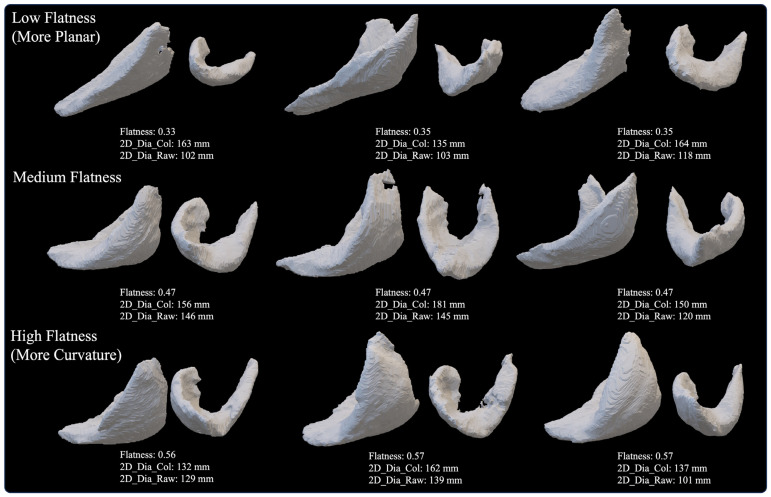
Representative Deltoid Muscle with Low (top row, more planar), Medium (middle row), and High (bottom row, more curvature) Values of Deltoid Shape Flatness for 3D Volumes Reconstructed from BONE Kernel CT Images.
